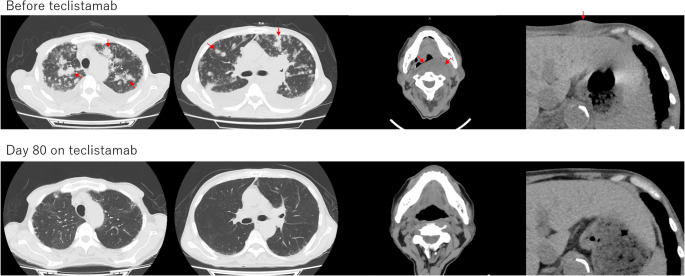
Radiological improvement of pulmonary and soft-tissue extramedullary lesions following teclistamab therapy. Computed tomography images before teclistamab initiation and on day 80 after treatment initiation. Red Arrows indicate multiple pulmonary nodules, the left posterior pharyngeal wall lesion, and the left abdominal subcutaneous mass. Multiple pulmonary nodules and soft-tissue extramedullary lesions evident at baseline showed marked regression, with complete radiologic resolution observed by day 80
